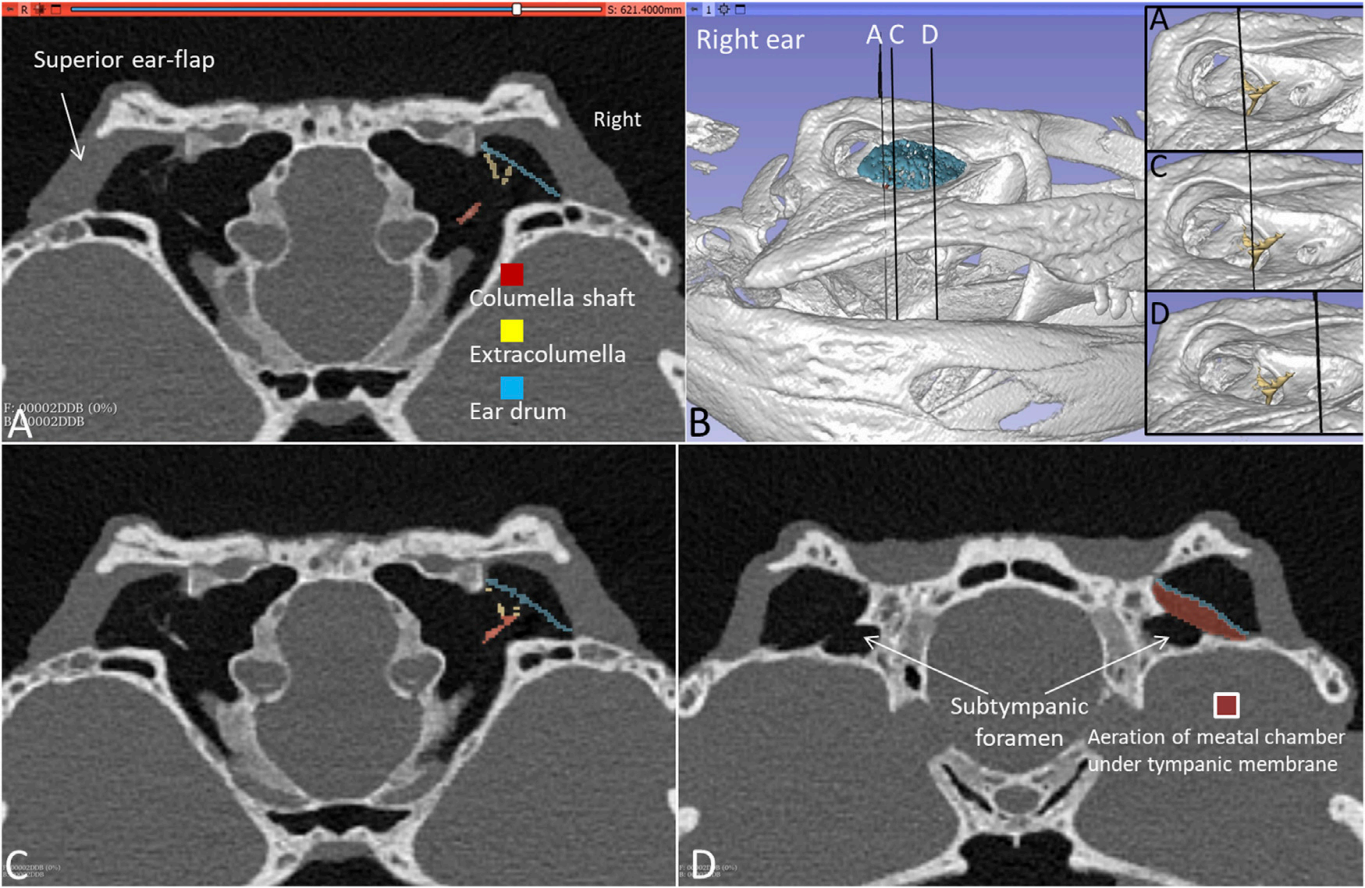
(A) PCD-CT with 3D rendering and segmentation demonstrating the aeration of the meatal recess in the living *C. rhombifer*. Section levels (A,C,D) of right ear are shown in (B) (insets without segmented ear drum). (A) Extracolumella touches the bony tympanic ring in right ear. The aeration of the meatal chamber underlying the tympanic membrane at the level of the subtympanic foramen is shown in (D).
